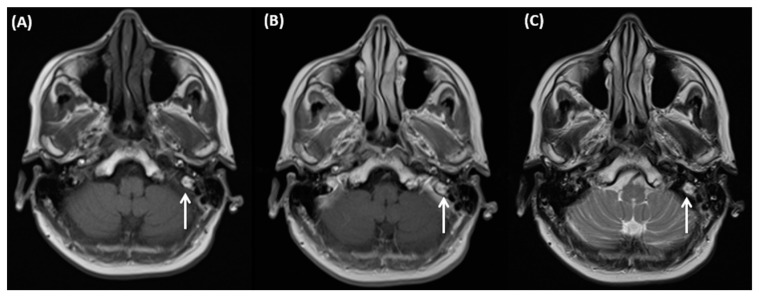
A 68-year-old female patient with incidental finding of a cholesterol granuloma located at the transition of the medial part to the apex of the left temporal bone (arrows). Axial T1-weighted MRI without (A) and with (B) administration of contrast agent. Axial T2-weighted imaging also shows the lesion as hyperintense (C).
